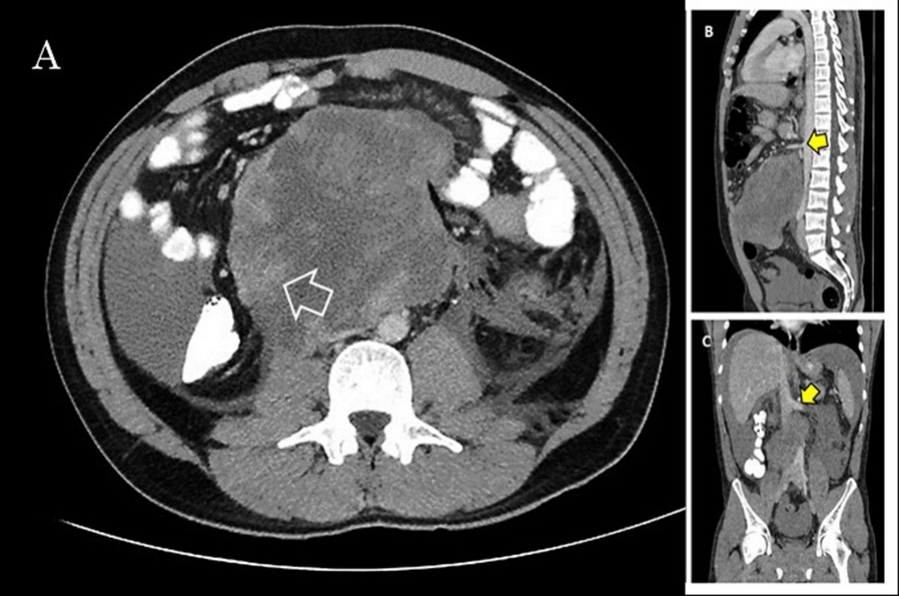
Preoperative abdominal contrast-enhanced CT showing A the large 13 × 11.4 × 15 cm retroperitoneal mass extending to the preaortic region below the renal veins. A liquefied and necrotic center with hyperdense areas inside the lymph node metastatic mass is clearly depicted (marked with a white arrow) on preoperative CT suggesting bleeding. B Sagittal view of the tumor and its relations with the superior mesenteric artery (yellow arrow) and C coronal view of the tumor and its relations with the left renal vein (yellow arrow), in addition to a significant amount of free intraperitoneal fluid suggestive of hematic content
